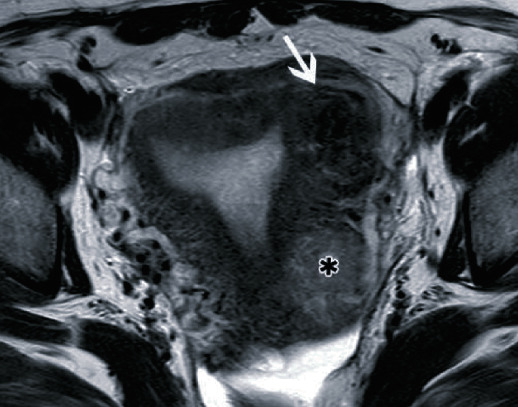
Magnetic resonance image 11 months following uterine artery embolization. T2-weighted axial image demonstrates further shrinkage of the residual fibroid (white arrow) and improvement of the endometrial irregularity. The asterisk (∗) shows another degenerative uterine fibroid.
